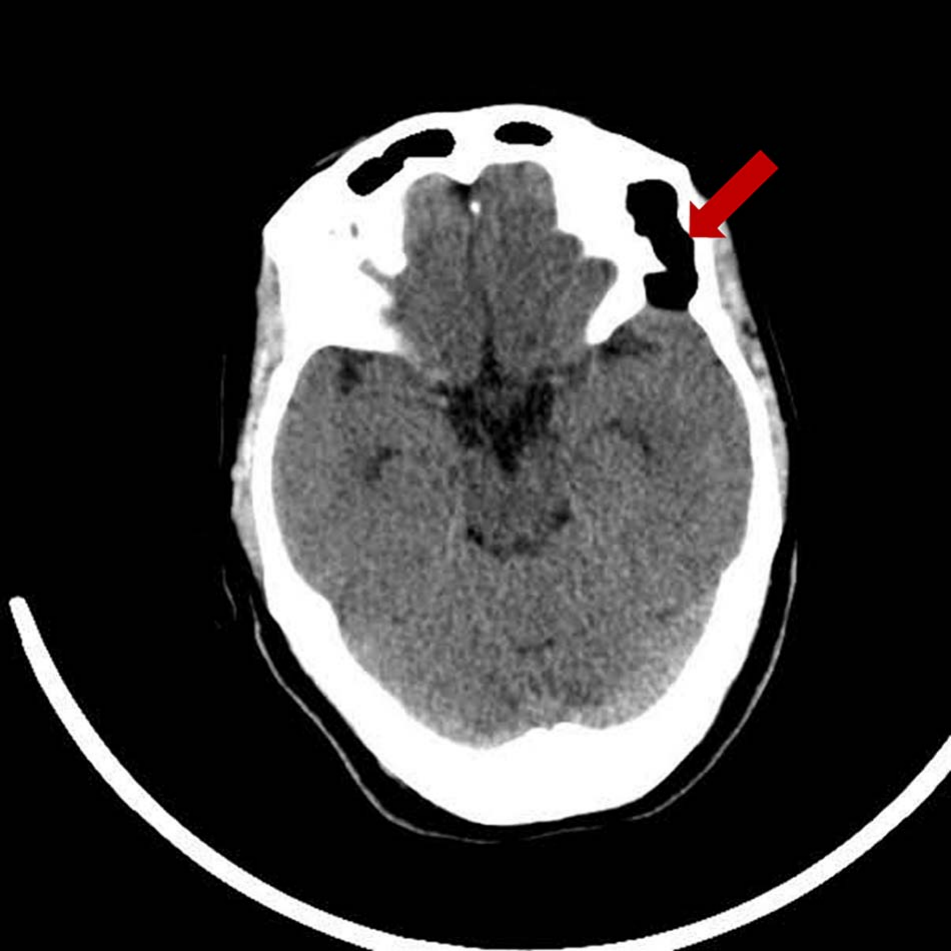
Pre-operative axial CT scan displaying a well-defined, low attenuating lesion in the left fronto-zygomatic region.
